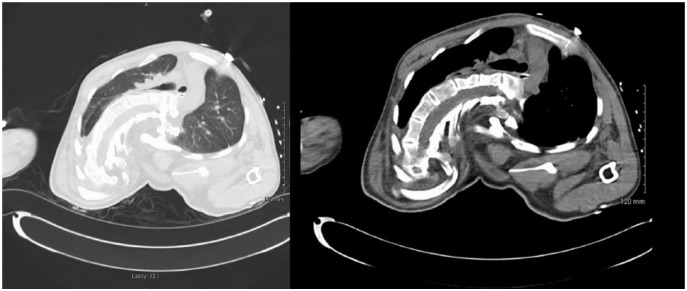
Axial computed tomography scan demonstrating severity of skeletal
